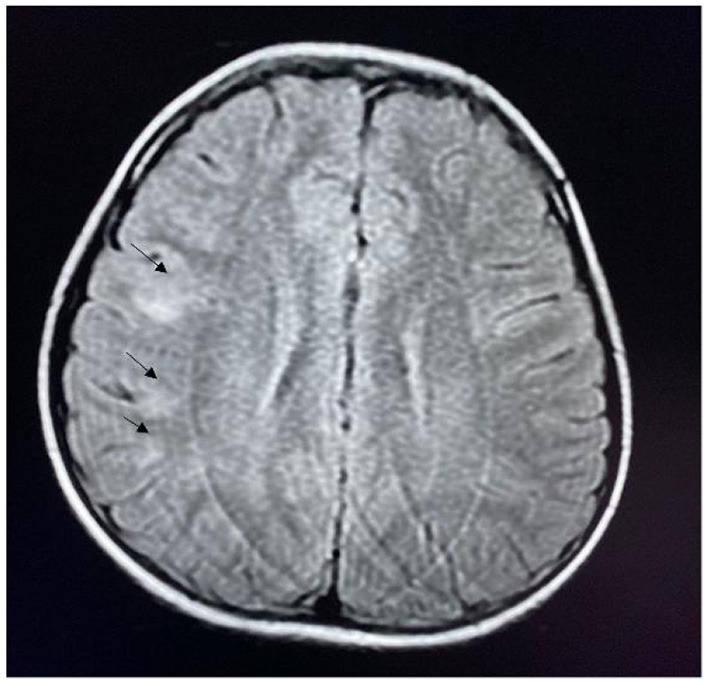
MRI of a patient with anti-NMDAR encephalitis showing lesions in the frontal and parietal cortex (arrow).
